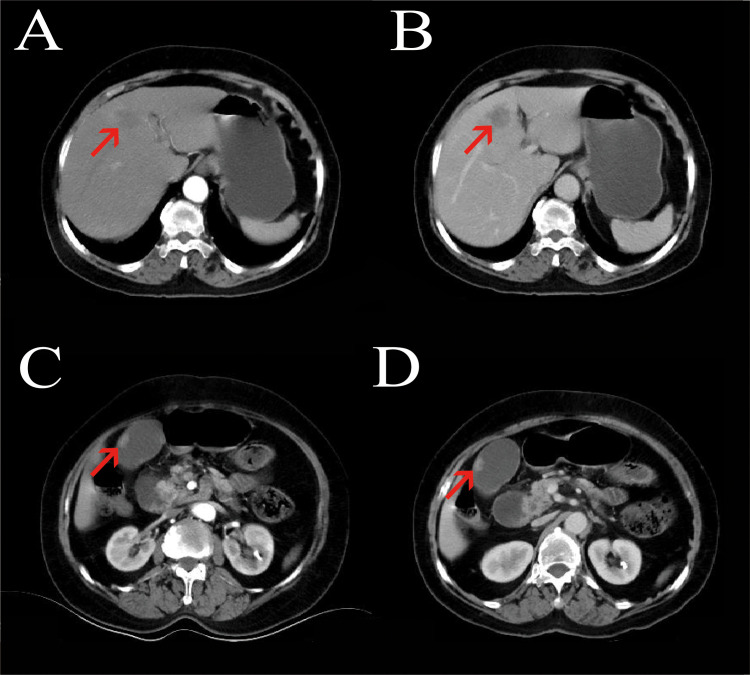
Contrast-enhanced abdominal CT. (A) Hepatic arterial phase; (B) Hepatic venous phase; (C) Gallbladder arterial phase; (D) Gallbladder venous phase.
